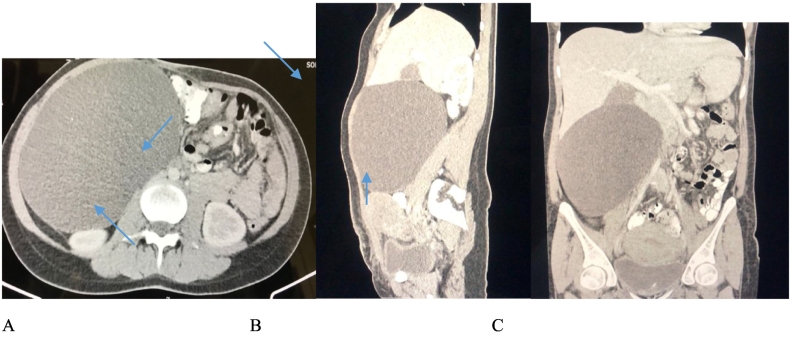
(A) Coronal view abdominopelvic computed tomography scan (CT scan) indicated a homogeneous unilocular cystic mass in the retroperitoneal space measuring about 20 × 15 cm with no enhancement and solid part in right quadrant of abdomen (B) sagittal view (C) axial view.
